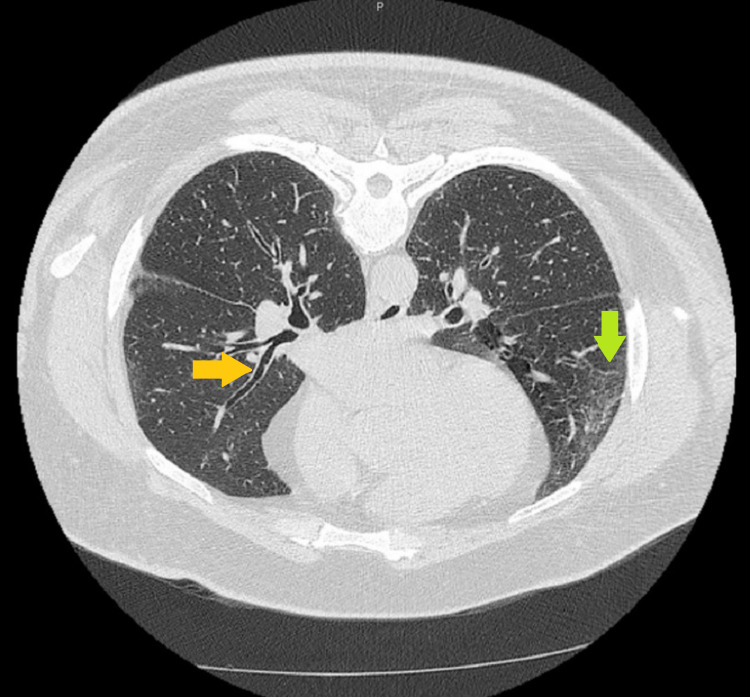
High-resolution CT scan showing a typical pattern of bronchiectasis with a tram track sign is seen in the image (orange arrow). Additionally, micronodules and non-specific ground-glass opacities are seen (green arrow).
